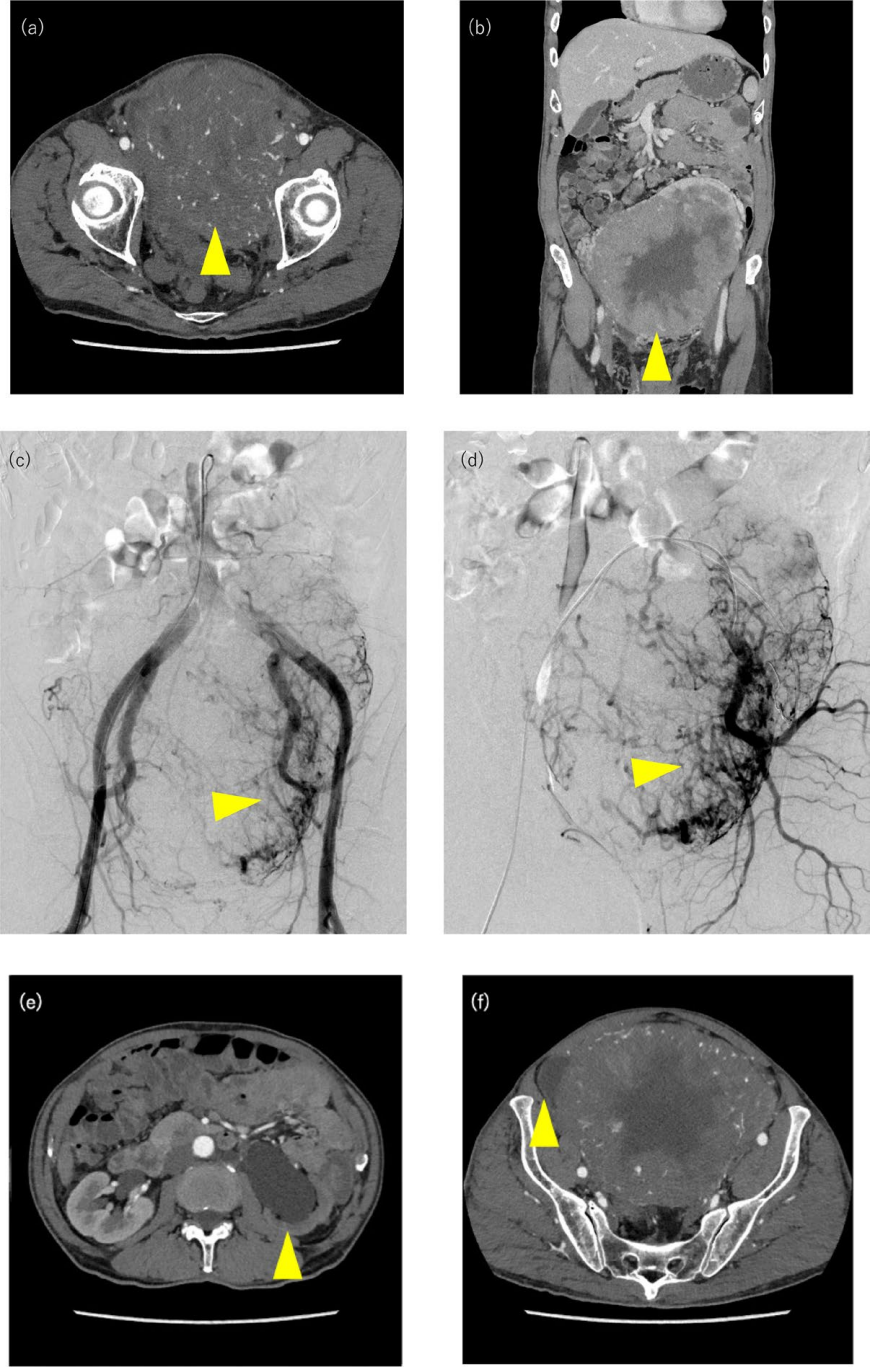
(a) Contrast‐enhanced CT showed enhancement of the tumor in a heterogeneous pattern. (b) A giant mass occupied in the pelvic cavity. (c, d) branches of the left internal iliac artery were the main feeders. (e) Left hydronephrosis developed due to ureteral obstruction. (f) Bladder was completely displaced to the right side.
